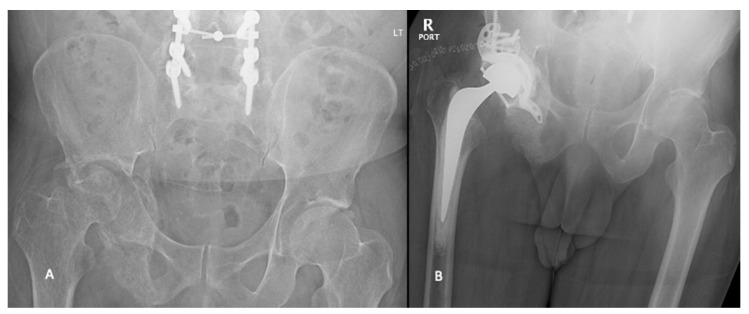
Pre- (A) and postoperative (B) radiographs of patient with metastatic small cell lung cancer who underwent cemented total hip arthroplasty with cage construct.
